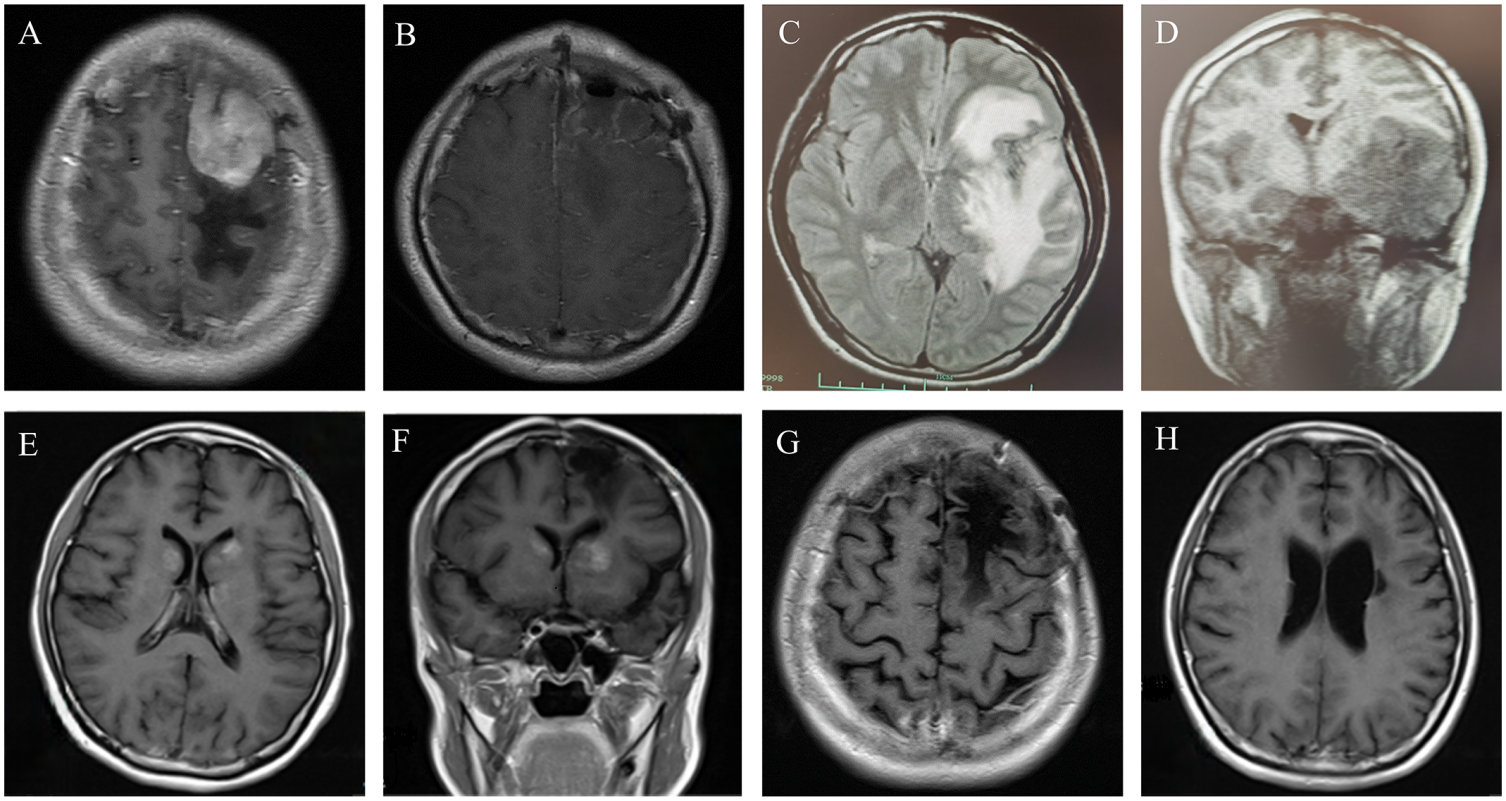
(A) Contrast-enhanced MRI revealed a 36*41*37mm homogenously enhancing lesion in the left frontal parietal lobe, with obvious space-occupying effect and peripheral edema. (B) Contrast-enhanced brain MRI after left frontal lobe tumor resection. (C, D) In September, 2019, the brain MRI showed the mass in the left frontal temporal lobe had clearly enlarged, considering relapse. (E, F) Axial T1 weighted image with gadolinium enhancement in February 2021, showing the patch−shaped enhanced masses in the bilateral basal ganglia. (G, H) The latest contrast-enhanced brain MRI showed that the masses in the bilateral basal ganglia disappeared.
